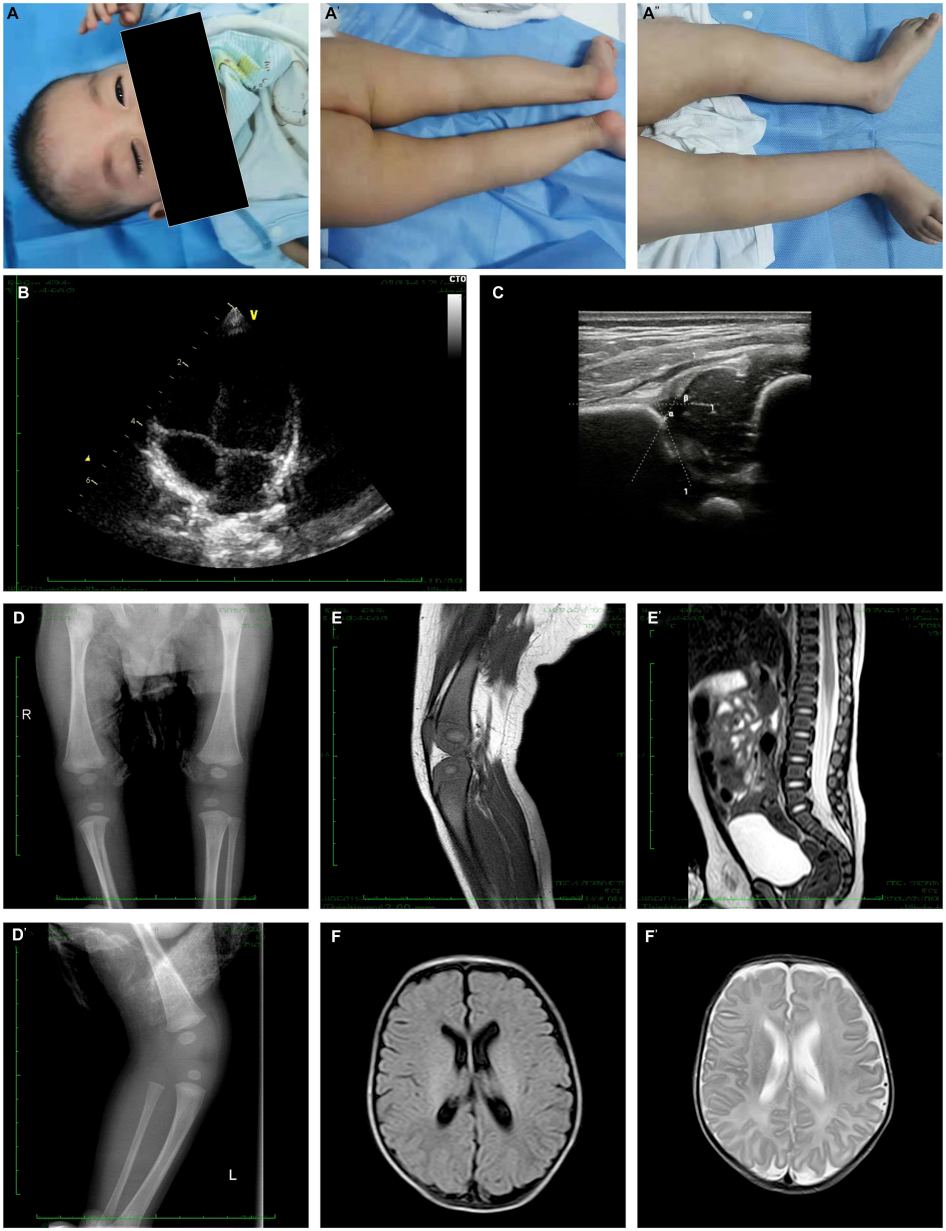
Clinical and radiology manifestation in the current proband. (A) The proband presented bilateral ptosis and impaired flexion of bilateral knees. (B) Echocardiography demonstrated a normal heart function and structure. (C) Hip ultrasonography showed Graf type I structure on his left hip joint. (D) X-ray examination of hip joint and knee joint showed that there was no definite abnormality in anterior-posterior view, while the lateral view demonstrated an over-extensive positive of knee joint. (E) The joint MRI presented a normal signaling in muscles, bones and ligaments associated to his knee joints while it still presented an over-extensive position, and the tail vertebras were normal. (F) The cerebral MRI revealed a normal structure and signaling both in T1 and T2.
